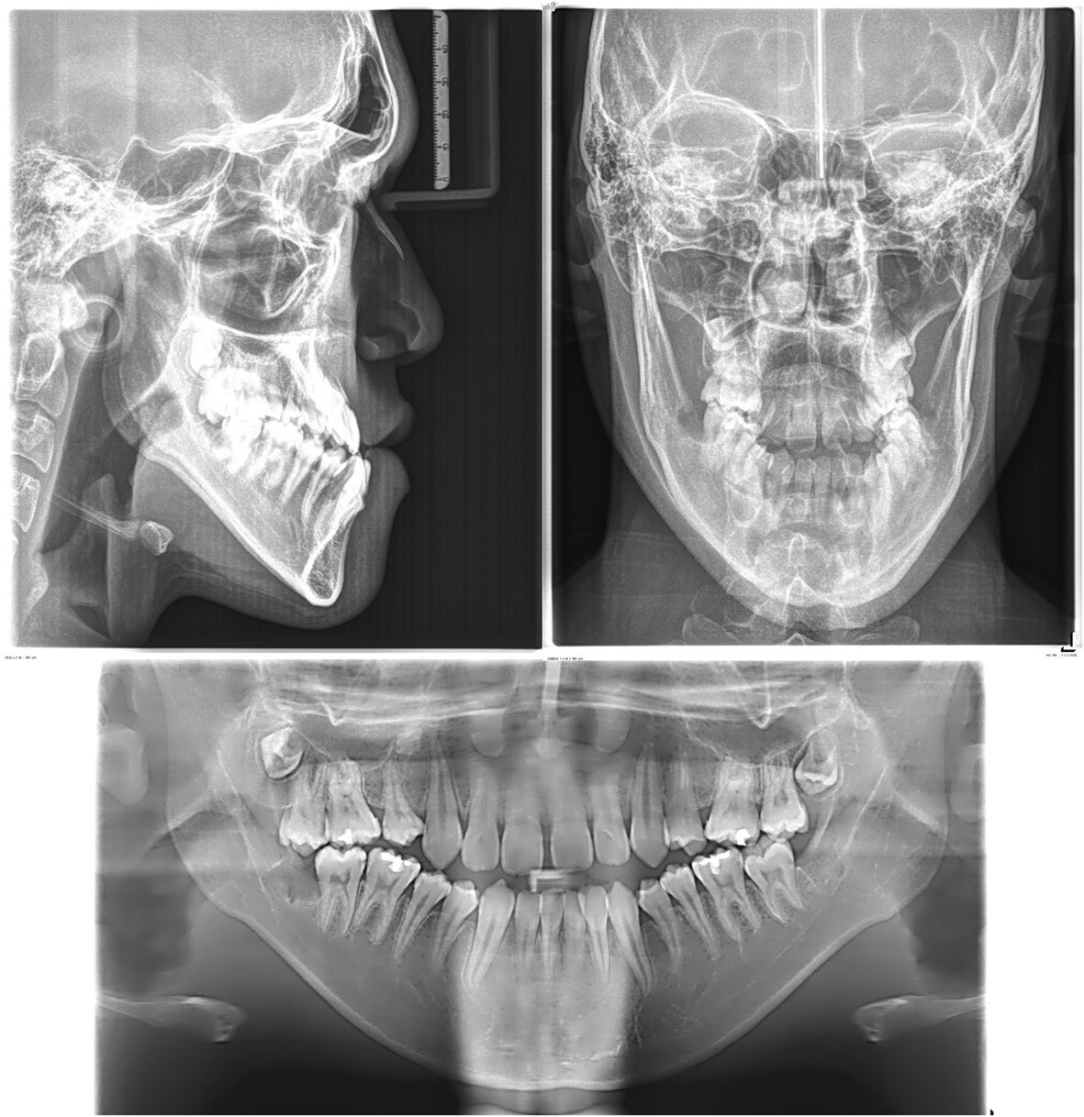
Initial lateral cephalometric, posteroanterior cephalometric, and panoramic radiographs
